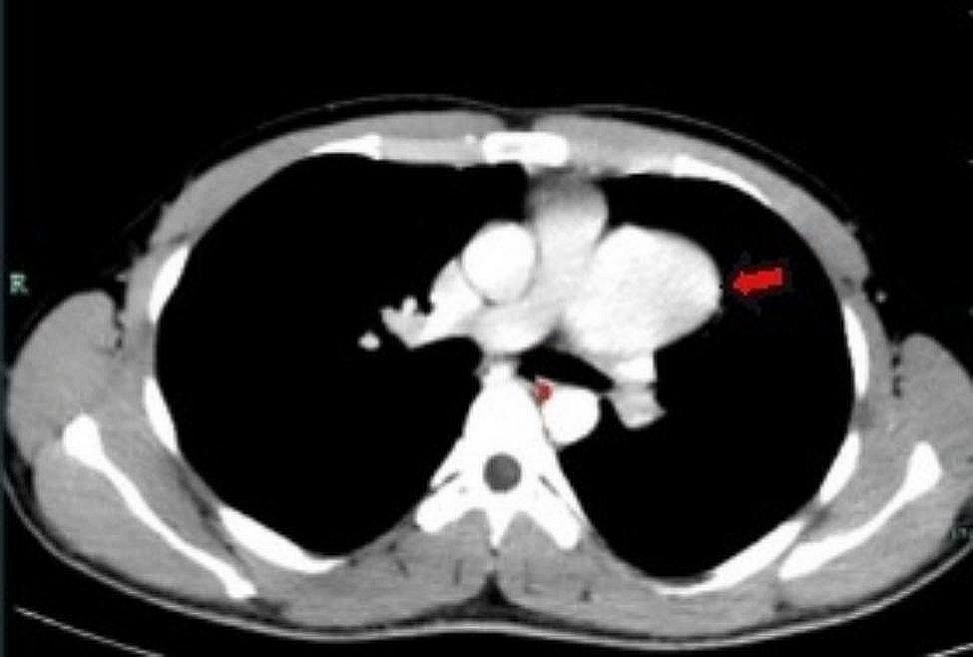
Chest-enhanced computed tomography
